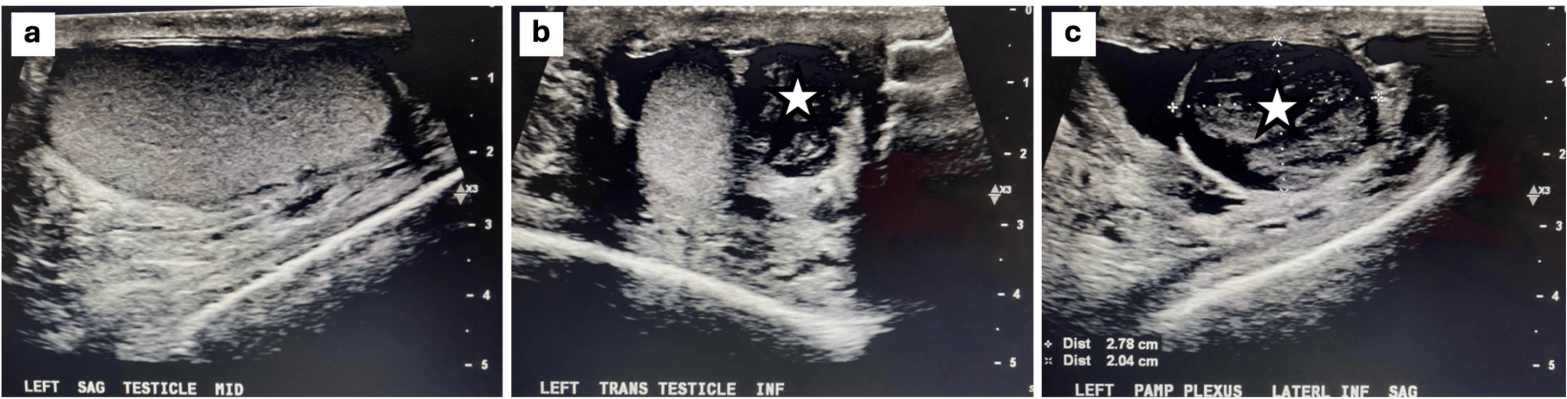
Scrotal ultrasound images upon presentation. (a) Sagittal mid view of the left testicle. (b) Transverse view of the left testicle and supratesticular mass. (c) Lateral inferior sagittal view of the left pampiniform plexus. This view also demonstrates the supratesticular mass. The star represents the supratesticular mass. All scale bars represent centimeters.
